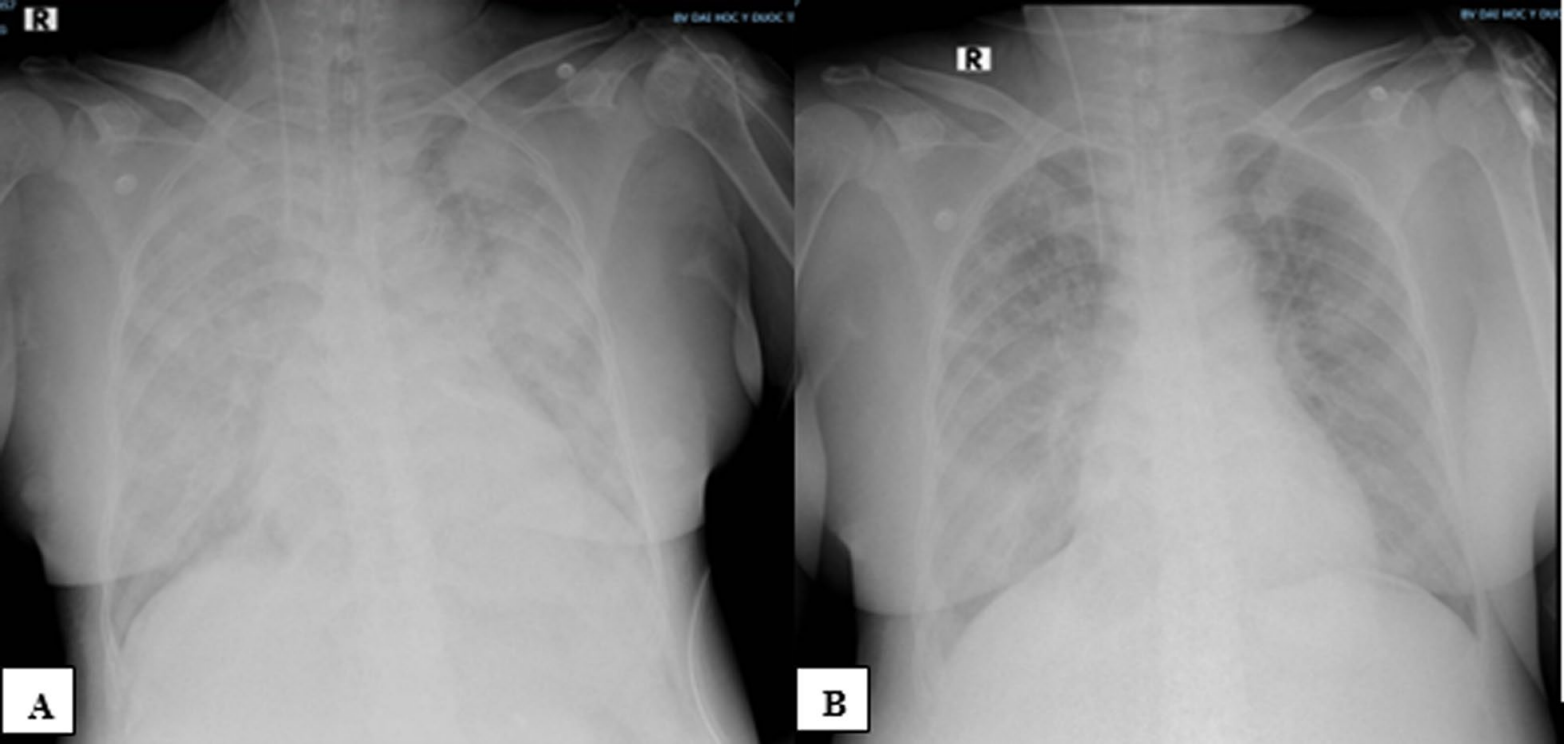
Changes in chest radiographs in the intensive care unit (ICU). A On the 1st day in the ICU, with worsening respiratory failure, the RALE score was 42. B On the 2^nd^ day, when the respiratory condition improved, the RALE score was 30
